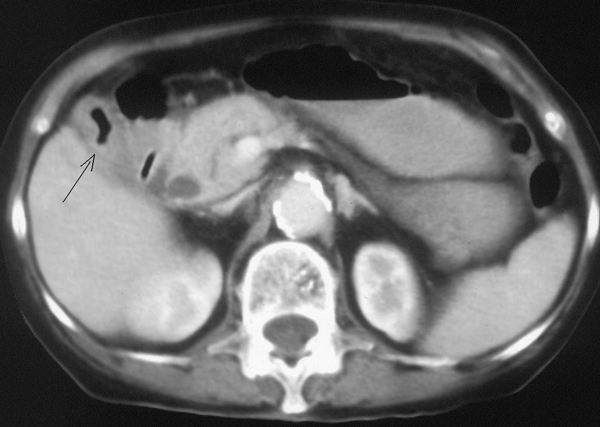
CT scan case 1: Gallbladder with thickened walls and with hydrogaseous levels.
